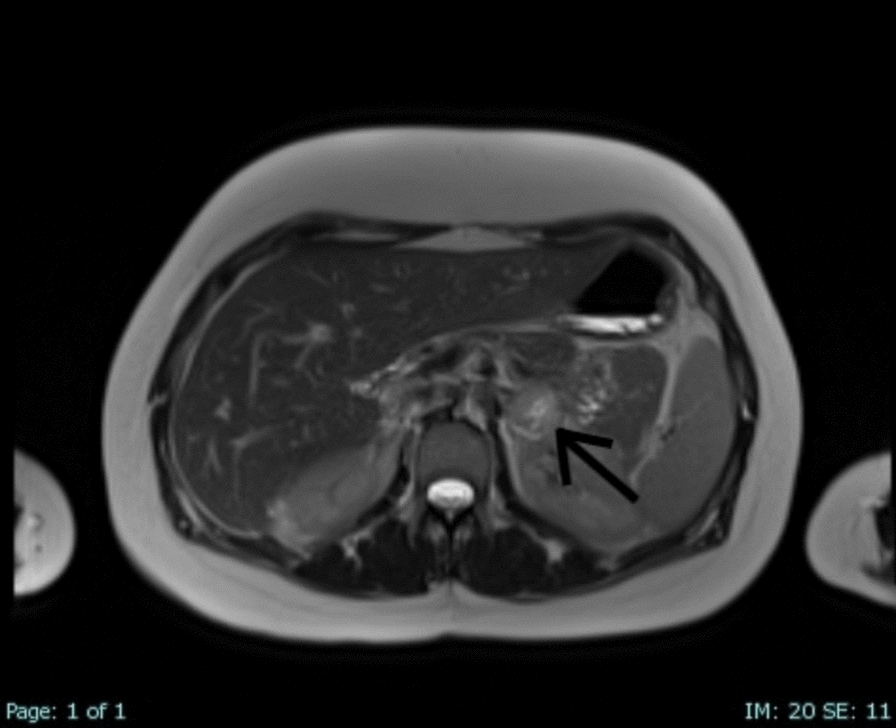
Postoperative dynamic abdominal coronal magnetic resonance imaging showing a tumor lesion in the left adrenal gland, indicated by the black arrow.
